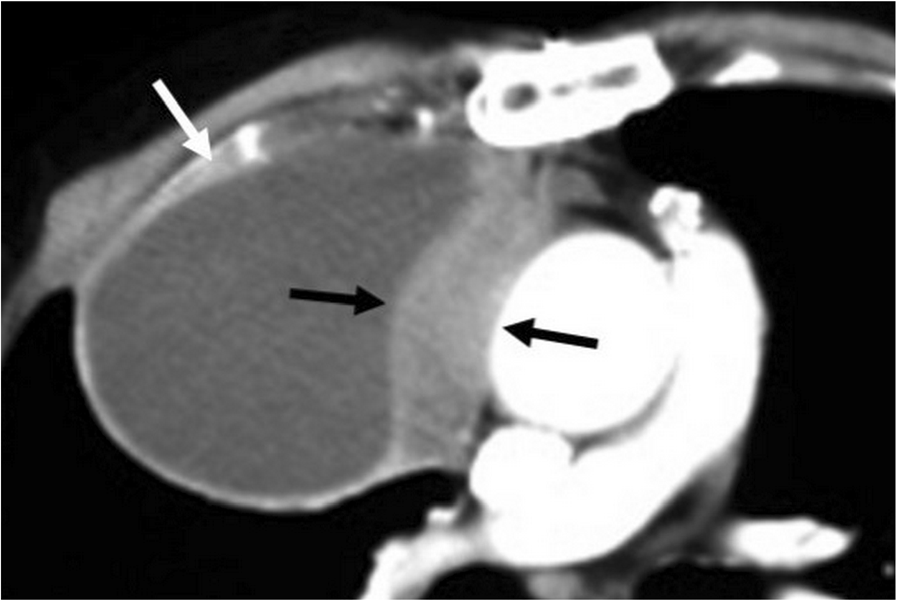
A 45-year-old man with pathologically proven pericardial mesothelioma. Axial contrast-enhanced CT image shows a heterogeneous pericardial mass with solid (black arrows) and cystic (white arrow) components. Note that there is a lack of fat tissue between the ascending aorta and the mass raising suspicion for perivascular invasion
